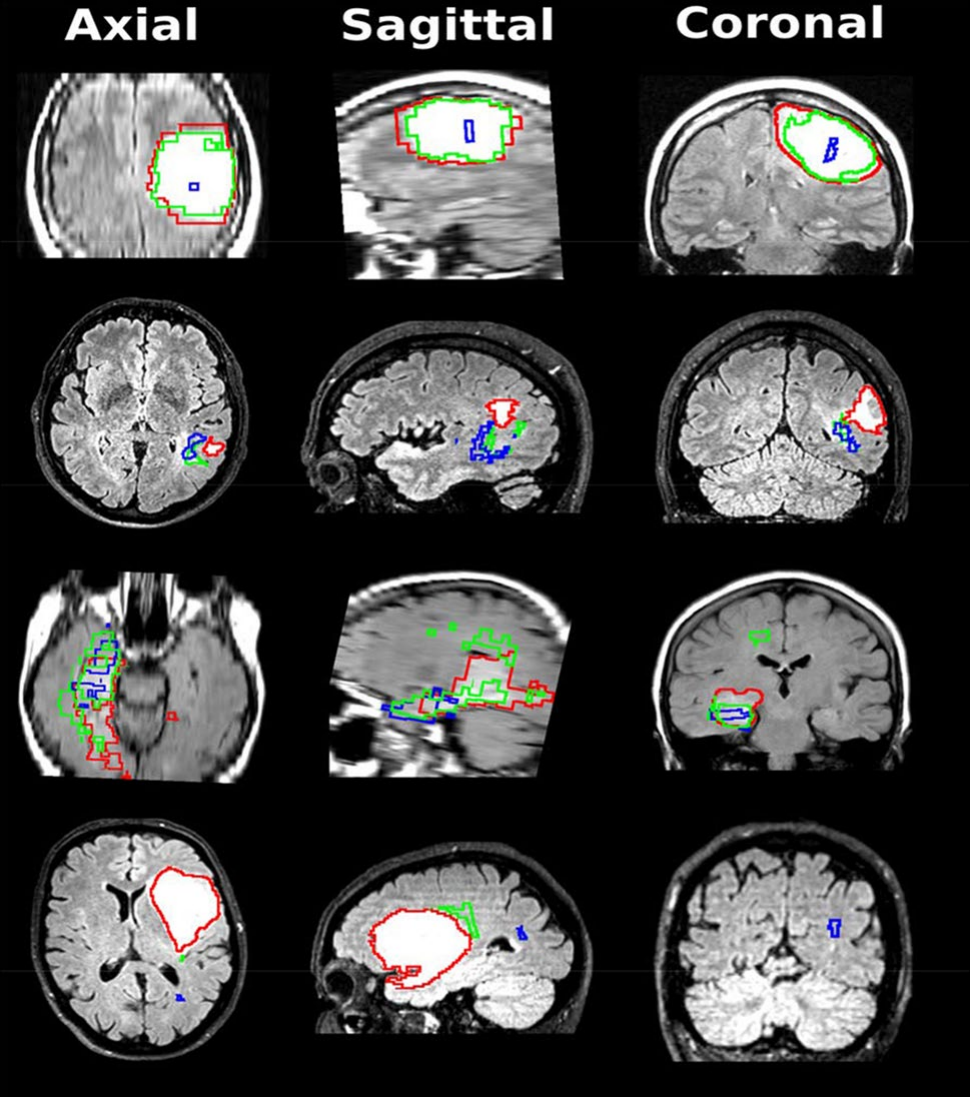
Different examples showcasing the pre-operative volume (in red), the pre-transformation volume (in green) and the transformation volume (in blue) on top of the pre-operative MRI volume (n = 35). Each row is representing a different patient, and each column is representing a different view. First row; local transformation within preoperative volume. Second row; local transformation without overlap. Third row; local transformation with border overlap. Fourth row; distant transformation
